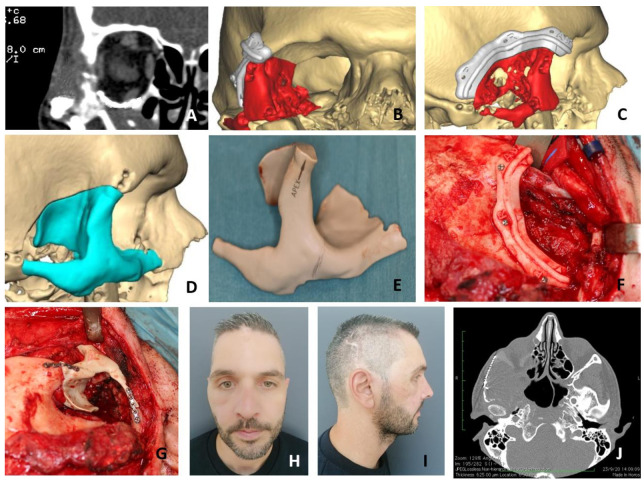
A 42-year-old male with a history of orbital floor fracture treated surgically with titanium mesh 7 years before being diagnosed during follow-up with a vascular malformation of the right zygomatic bone (A). Virtual surgical planning was performed for resection and reconstruction with a PEEK implant. Cutting guides were designed for resection (B,C). A customized PEEK PSI was specifically designed for the reconstruction (D,E). Due to the history of orbital floor surgery with a titanium mesh, it was decided to remove it and to extend the PSI to the orbital floor to avoid possible interferences between both reconstructions. A hemicoronal approach was performed and cutting guides were used to perform the resection (F). Once the malformation was removed, the PEEK PSI was placed with an excellent fit (G). The postoperative CT scan showed a correct placement of the PEEK PSI with an optimal aesthetic result (H–J).
